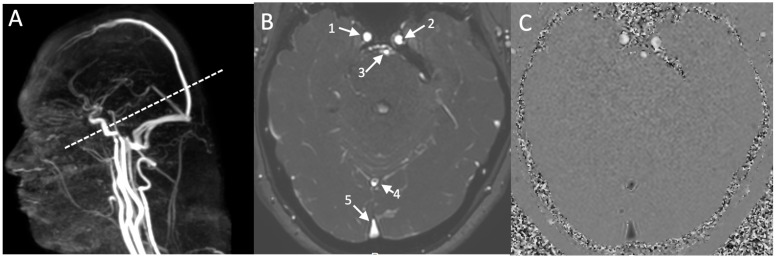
(A) Cross-sectional plane of vascular acquisitions by phase-contrast sequence MRI (PCMRI). PCMRI produced 32 amplitude images and 32 phase images in which pixel intensities are functions of the velocities of the blood. (B) One of the amplitudes images of the cardiac cycle: 1—right carotid artery; 2—basilar artery; 3—left carotid artery; 4—straight sinus; 5—superior sagittal sinus. (C) Phase image showing the arterial flows in white and the sinus flows in black.
